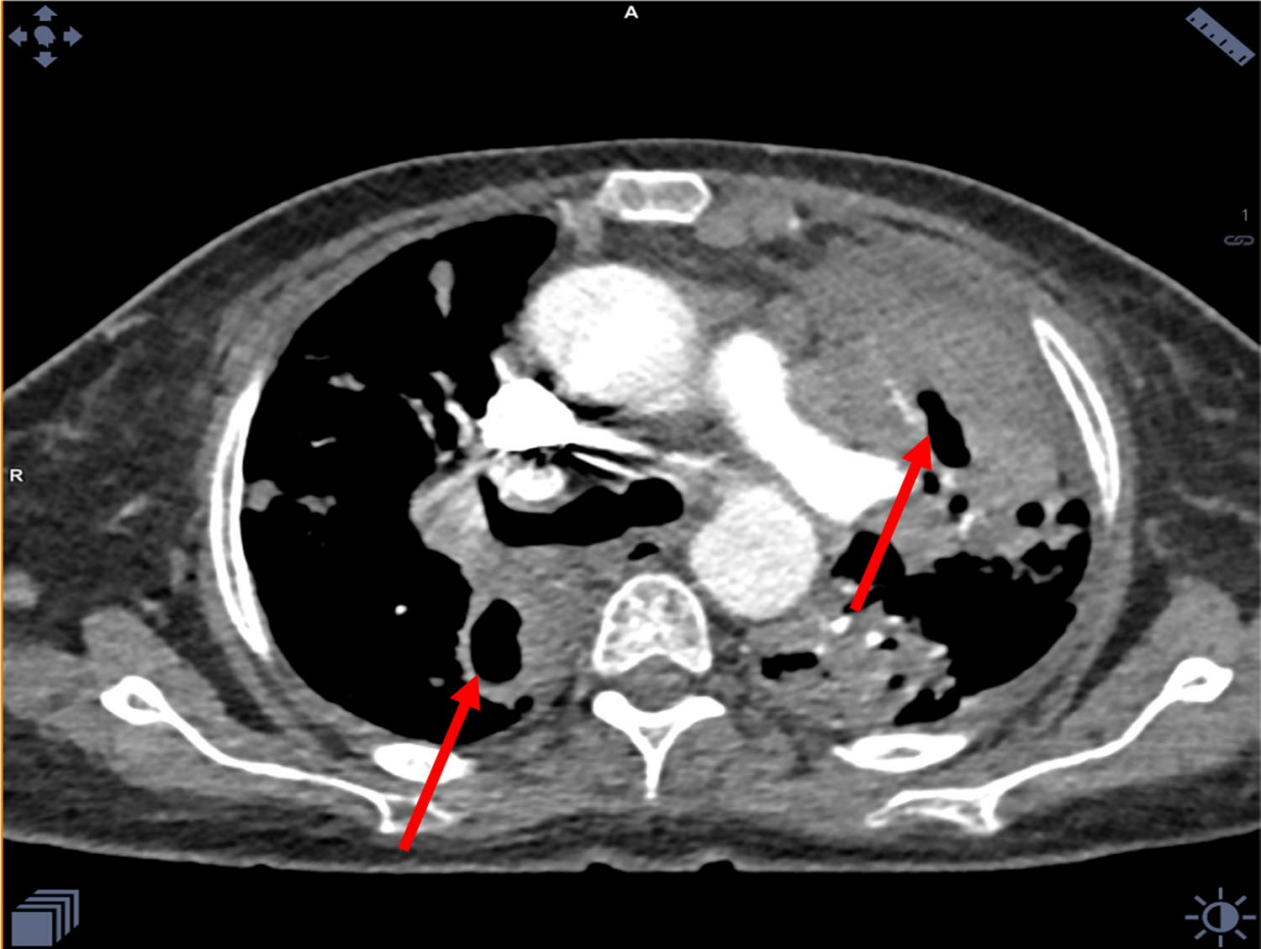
CT chest angiogram (lung window) showing consolidation with cavitary lesion bilaterally (red arrow)
